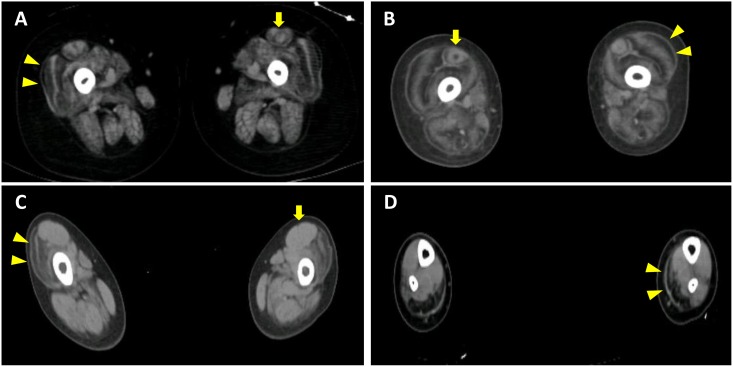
Muscle CT findings. For UCMD, the periphery of vastus lateralis was predominantly affected (arrowhead) with variable involvement of rectus femoris (arrow) (A from patient 7 and B from patient 8). For L-CMD, vastus lateralis was preferentially involved with hypertrophy of rectus femoris at thigh level (C), and medial head of gastrocnemius was predominantly affected at calf level (D).
